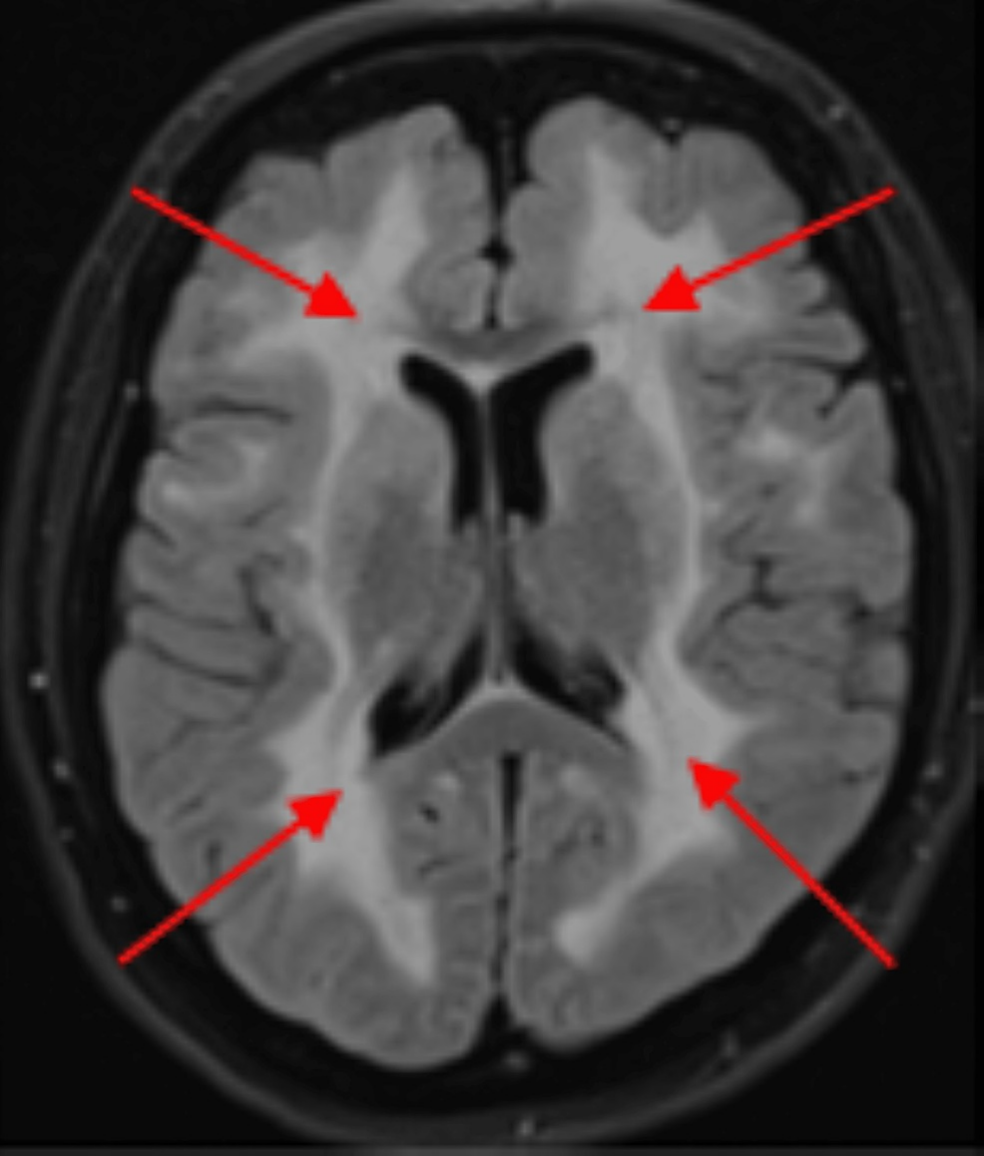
MRI FLAIR sequence showing extensive signal abnormality in the white matter (red arrows). FLAIR: fluid-attenuated inversion recovery.
